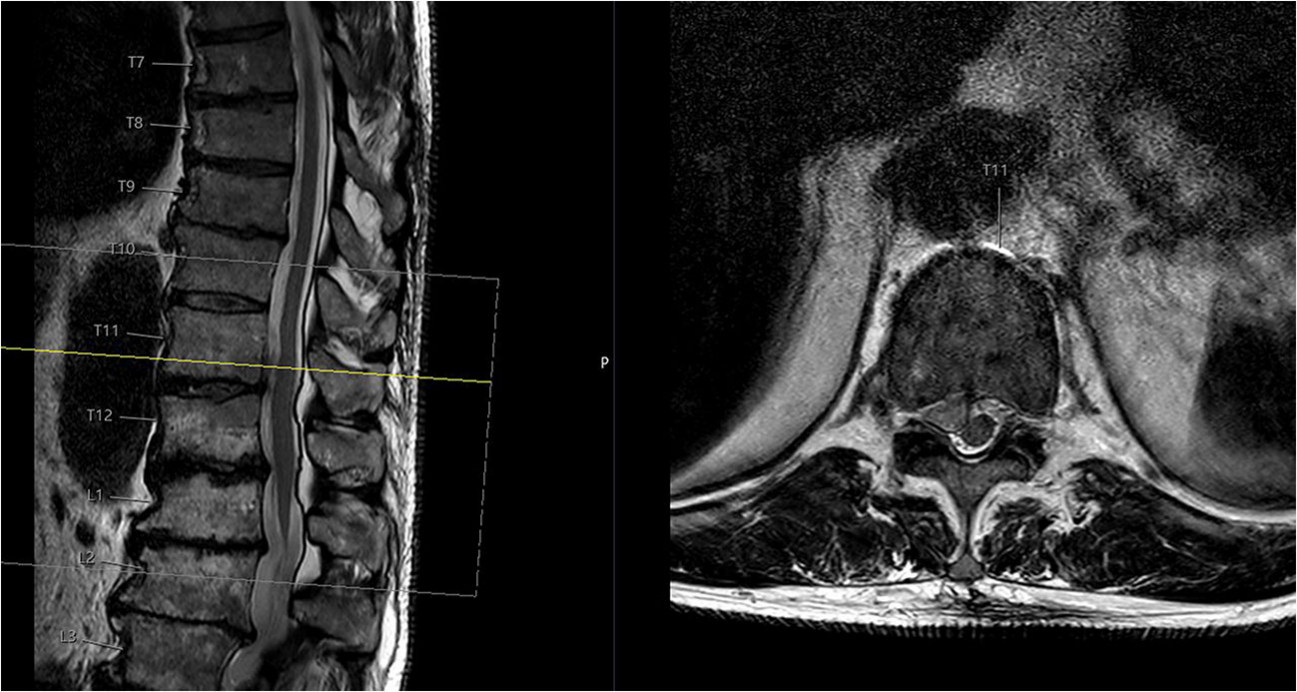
The MRI scan of the thoracolumbar spine T2 weighted axial and sagittal presents a right-sided disc herniation TH 11/12
